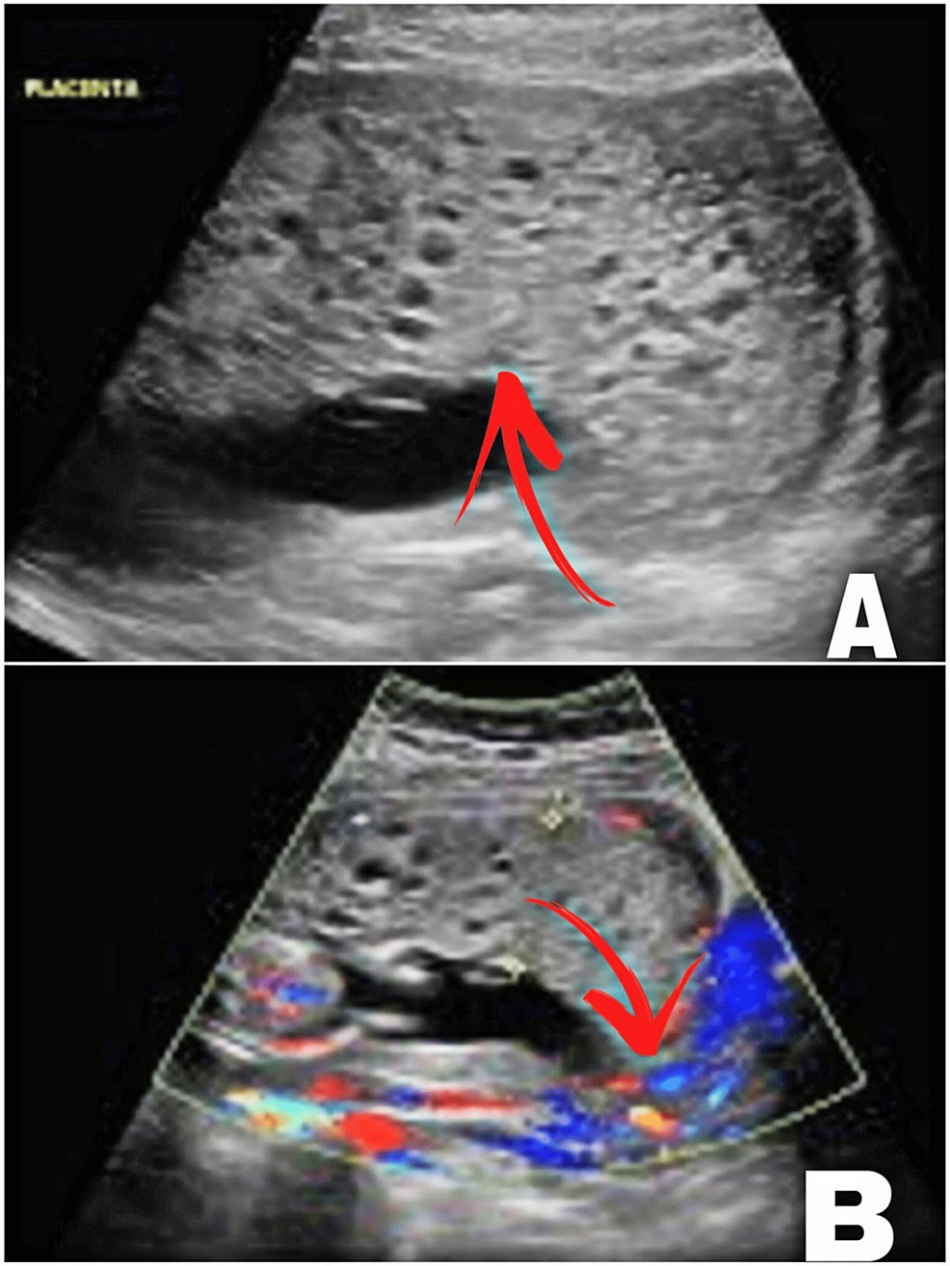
Ultrasound at 21 weeks revealed placental cystic changes
